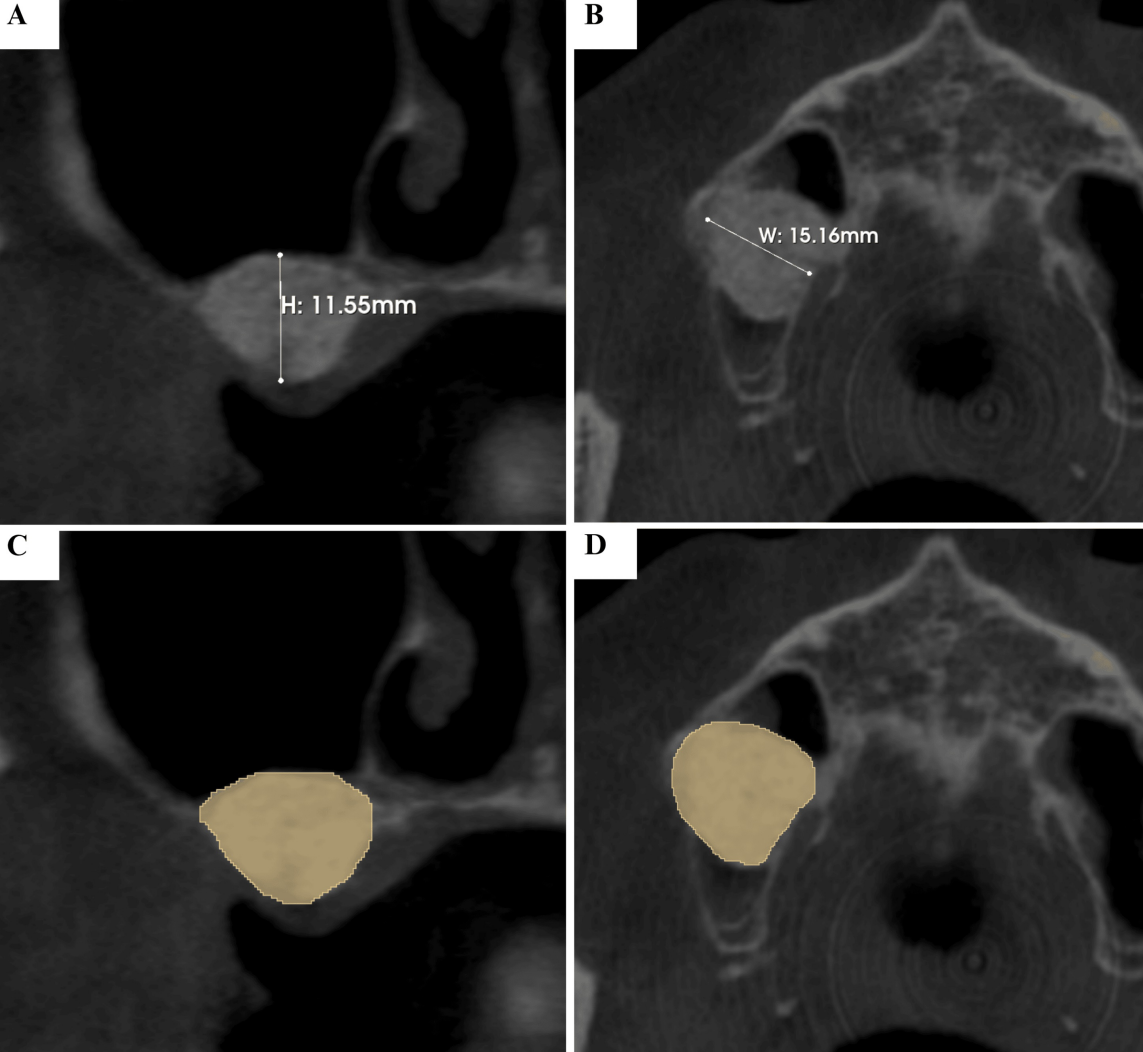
Cone-Beam Computed Tomography (CBCT) Analysis of the Grafted Area at T1 The grafted area at T1 showed a height of 11.55 mm and a width of 15.16 mm. Densitometric analysis revealed an initial mean Hounsfield Unit (HU) value of 297.24 HU, with an overall grafted bone volume of 1978.61 mm³. The highlighted region represents the grafted area within the maxillary sinus, from which linear, volumetric, and CBCT-based densitometric measurements were obtained. (A) Sagittal CBCT slice showing the vertical height of the grafted area (11.55 mm). (B) Axial CBCT slice demonstrating the horizontal width of the grafted area (15.16 mm). (C) Sagittal view with segmentation of the region of interest (ROI) for volumetric and densitometric analysis. (D) Axial view with ROI segmentation used for three-dimensional volume assessment.
